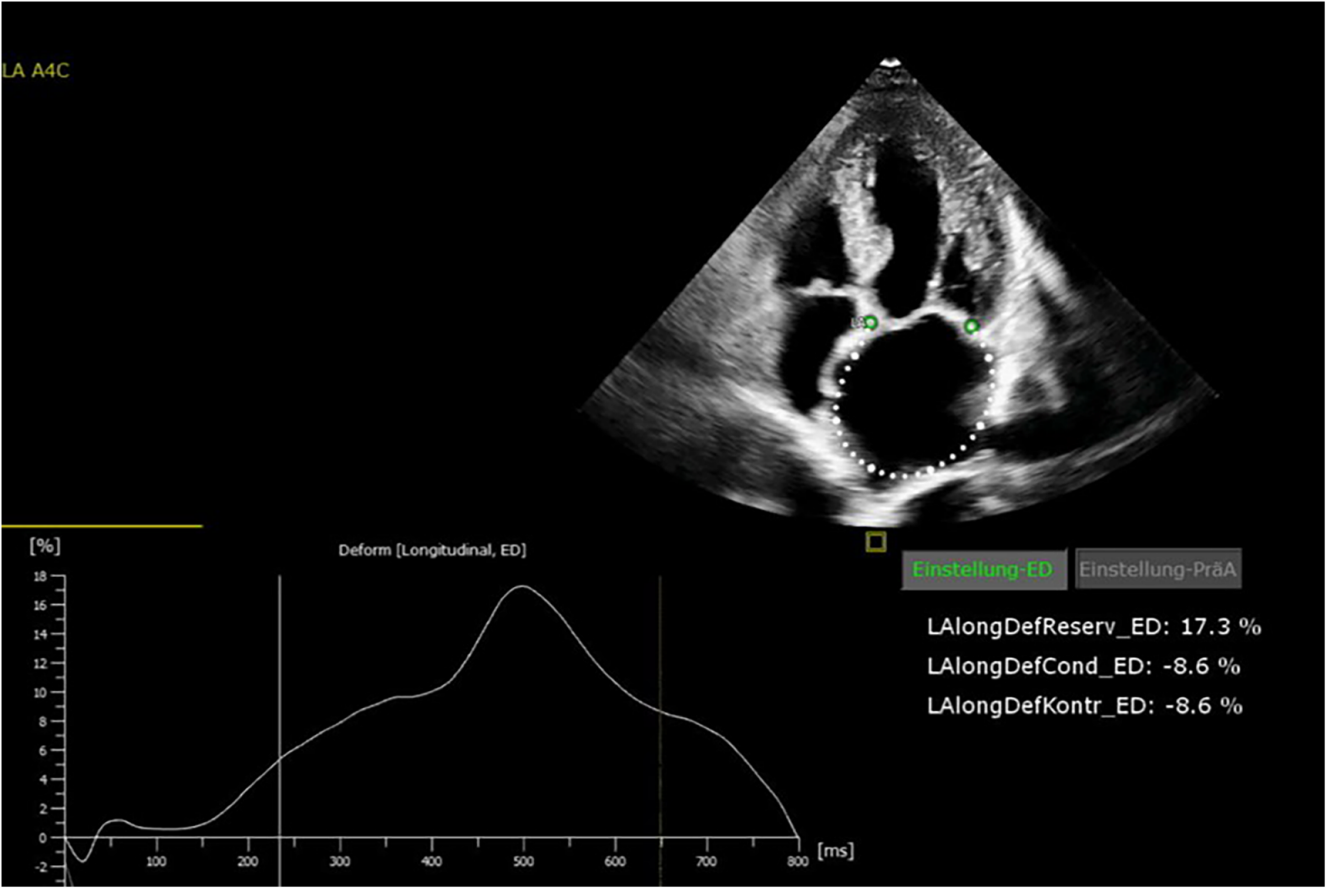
Echocardiography showing reduced left atrial (LA) strain values.
